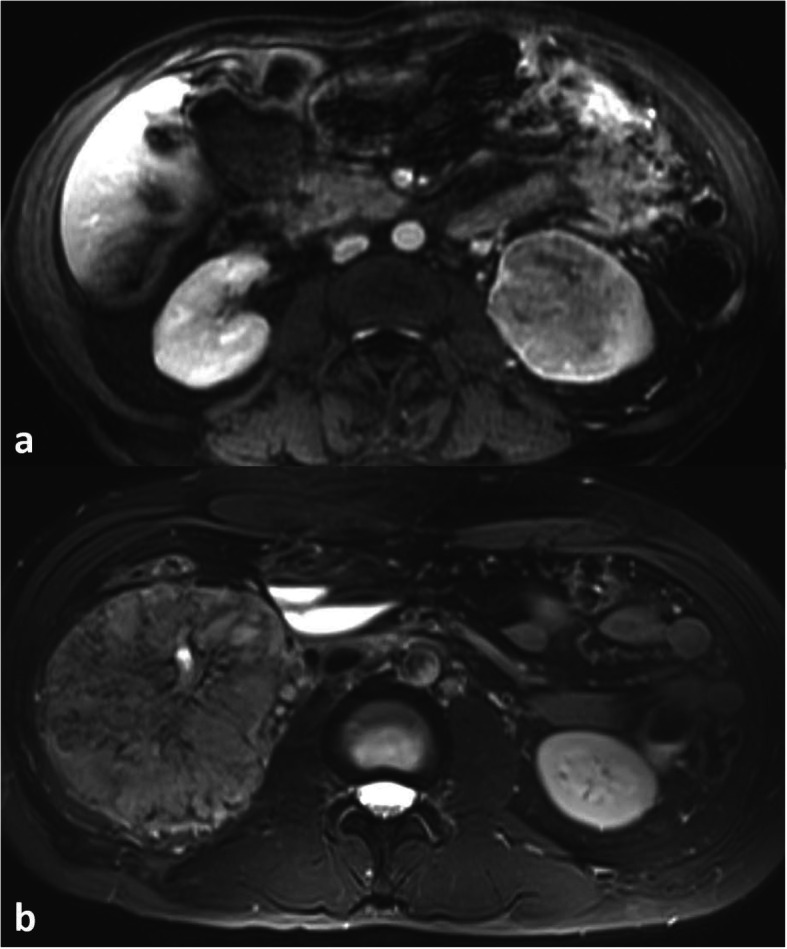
38-year-old male with fumarate hydratase-deficient renal cell carcinoma (FH-RCC). On magnetic resonance imaging, axial post-contrast 3D fat-saturated T1-weighted sequence showing a left renal mass with heterogenous enhancement (a). 63-year-old male with FH-RCC, axial fat-suppressed T2-weighted sequence showing a right renal mass with heterogenous T2 signal (b)
